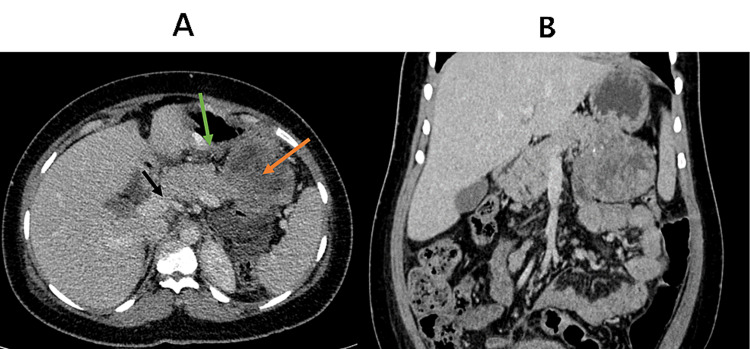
(A) and (B) CT scan of the abdomen (axial and coronal views) showing a heterogeneous mass with a mixture of solid and cystic components as well as multiple foci of calcification abutting both the tail of the pancreas and the stomach
